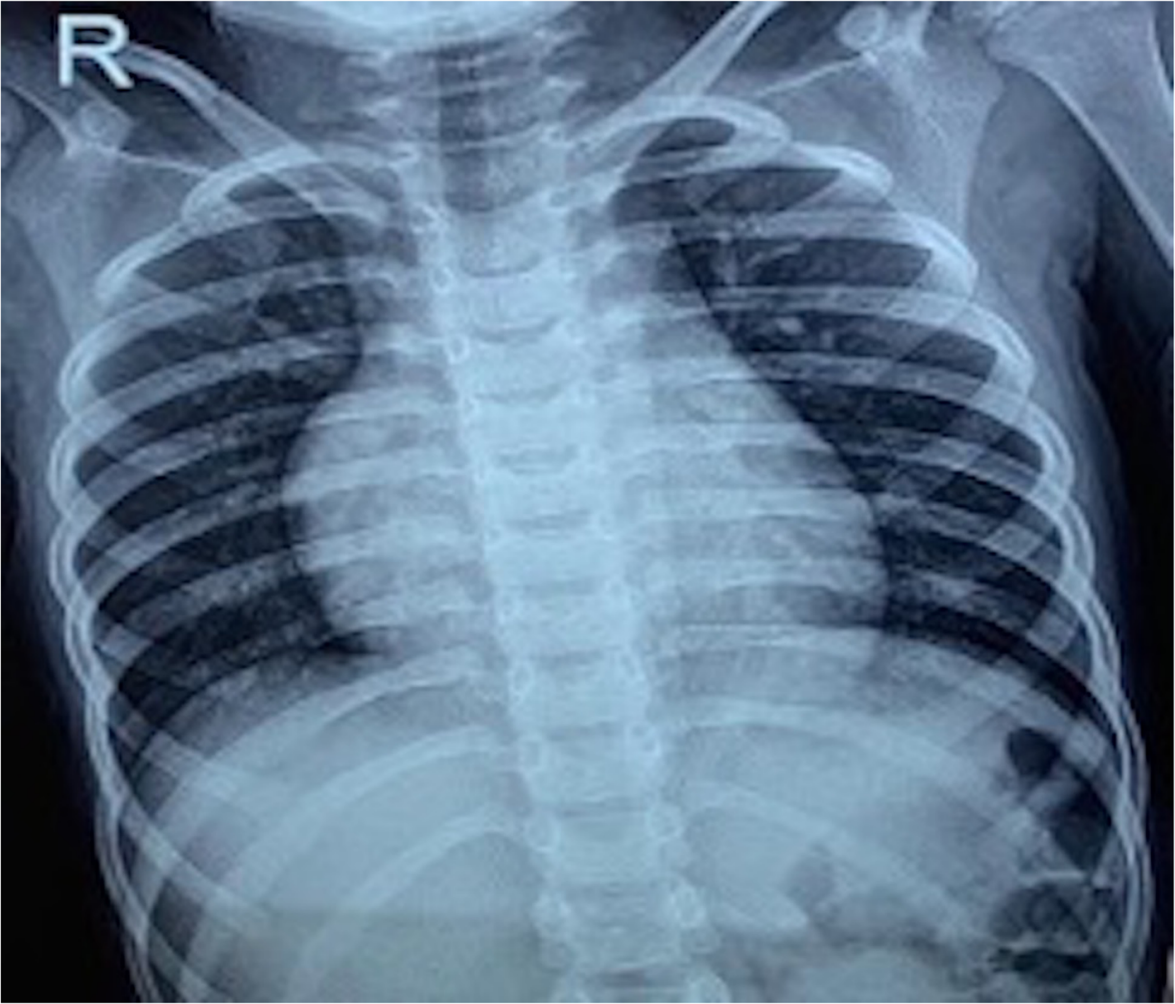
Chest x-ray showing right atrial, pulmonary arterial enlargement with cardiomegaly and right ventricular apex. Numerous end-on vessels are seen over bilateral lung fields suggestive of increased pulmonary blood flow
